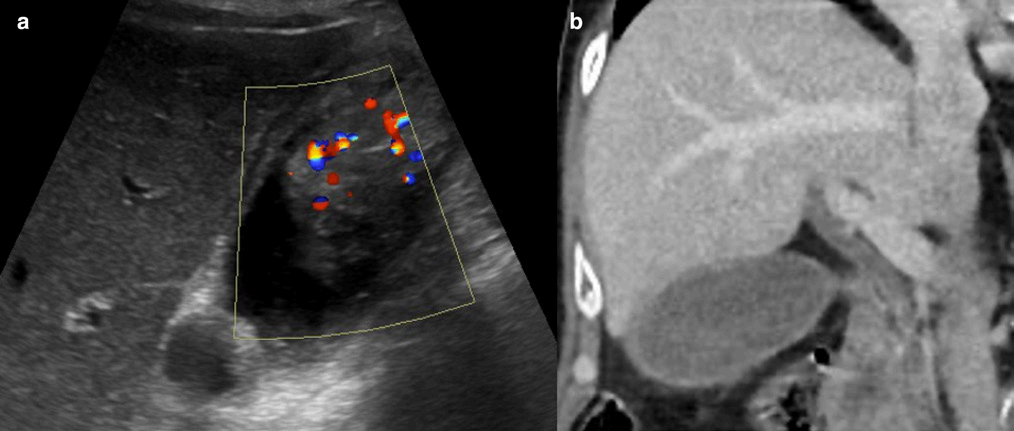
(a) Greyscale ultrasound images in longitudinal section demonstrating a “polypoid mass” with twinkling artefact on Doppler assessment. The twinkling artefact is thought to result from the presence of either calcifications or cholesterol deposits and it mimics high-velocity blood flow which can raise concern for a soft tissue lesion. (b) Subsequent coronal contrast-enhanced CT demonstrates sludge in the gallbladder lumen, appearing mildly bright with intact gallbladder wall and no evidence of a mass.
